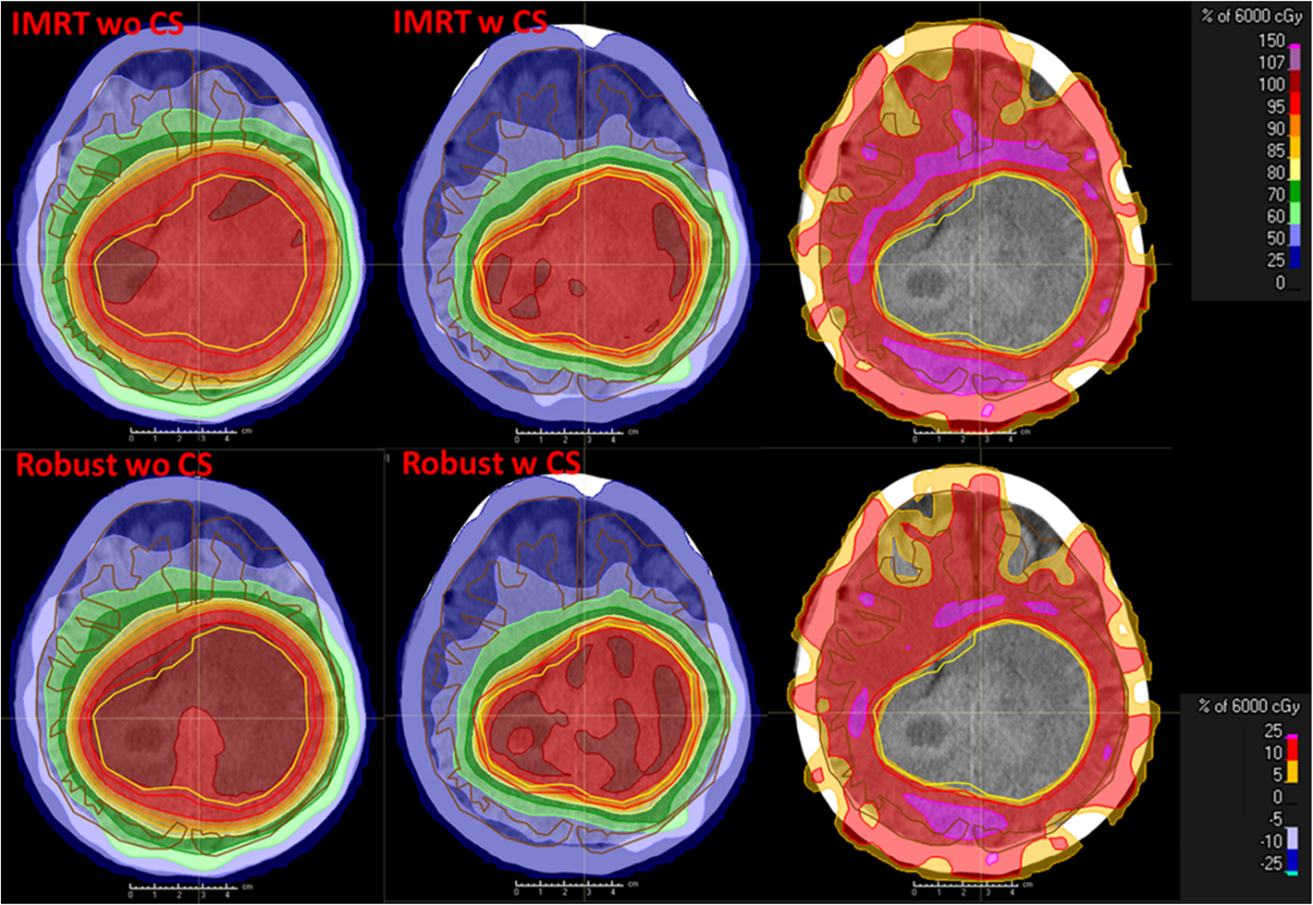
IMRT and robust dose distributions for patient B on a representative CT slice. Left column: IMRT/ robust plan without cortex sparing (wo CS). Middle column: IMRT/ robust plan with cortex sparing (w CS). Right column: dose difference (plan wo CS minus plan w CS). The dose difference shows the potential of cortical sparing of up to 25% (purple area) for this slice. Red and yellow areas indicate a dose reduction of 10%, and 5%, respectively
